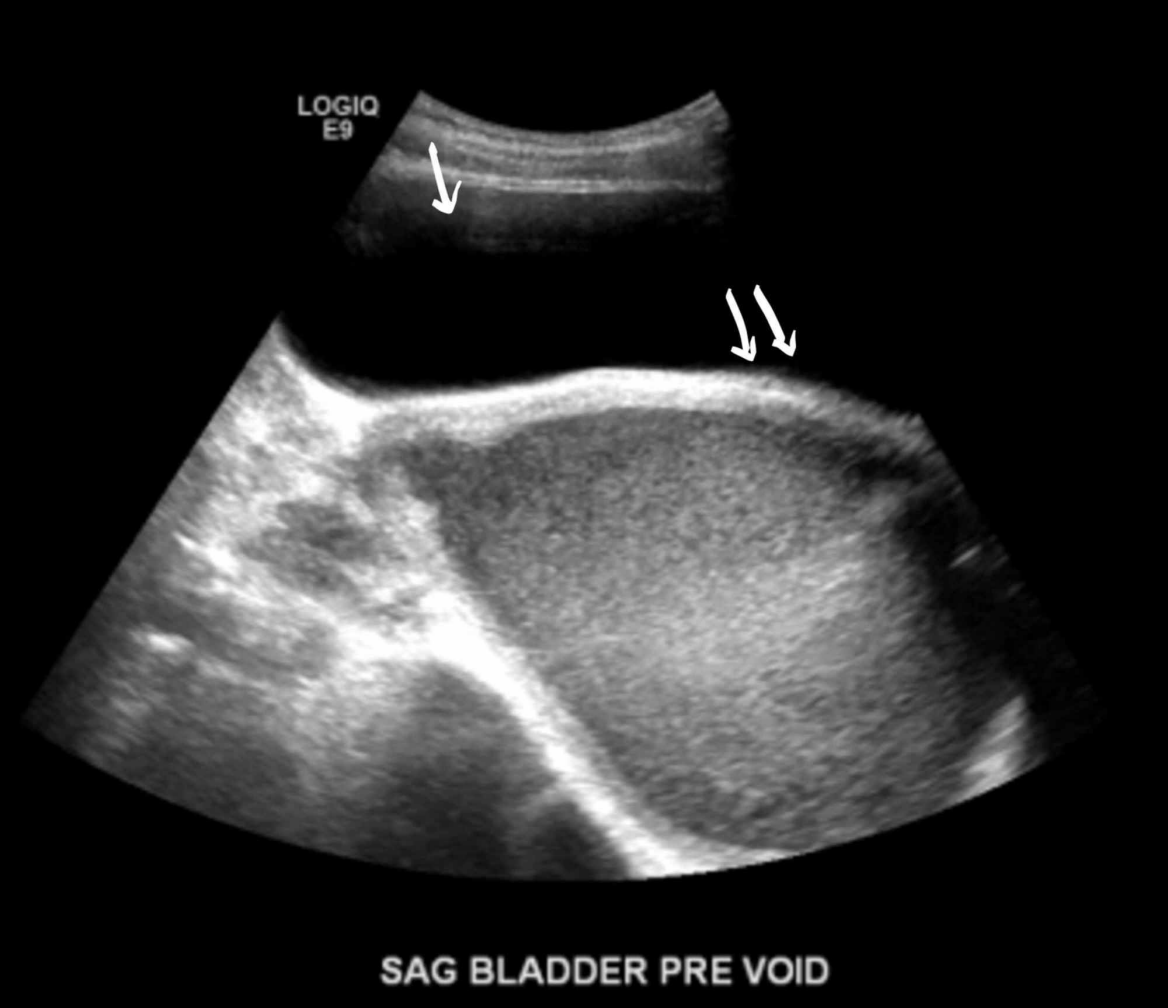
Ultrasound of bladder. Single arrow: significant postvoid urinary bladder residual volume of 264 ccs. Double arrows: well-defined structure of uncertain etiology posterior to the urinary bladder with a dominant component measuring approximately 12.2 cm.
